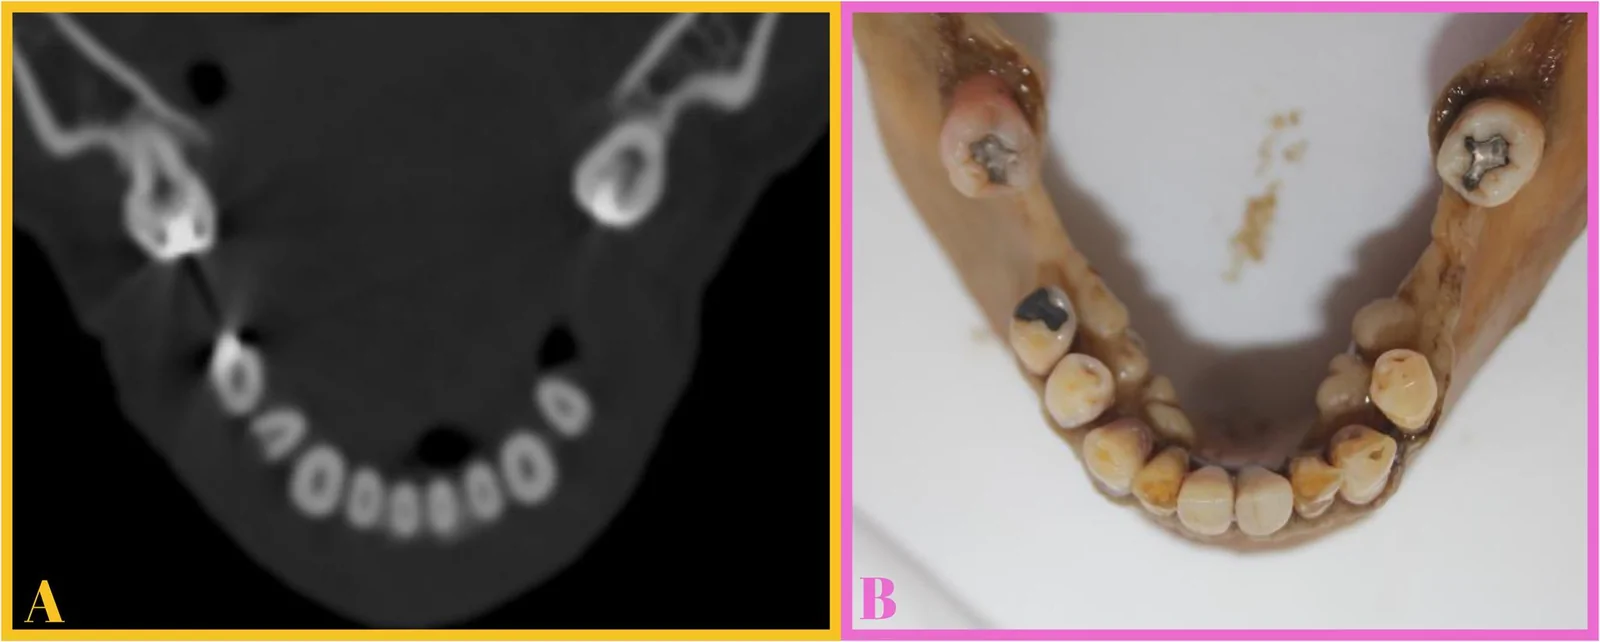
Forensic odontological comparison between an axial CT slice of the mandible and the occlusal view of the mandibular arch, showing morphological compatibility as well as correspondence between present and absent teeth, their rotational position, and the presence of silver amalgam restorations.
